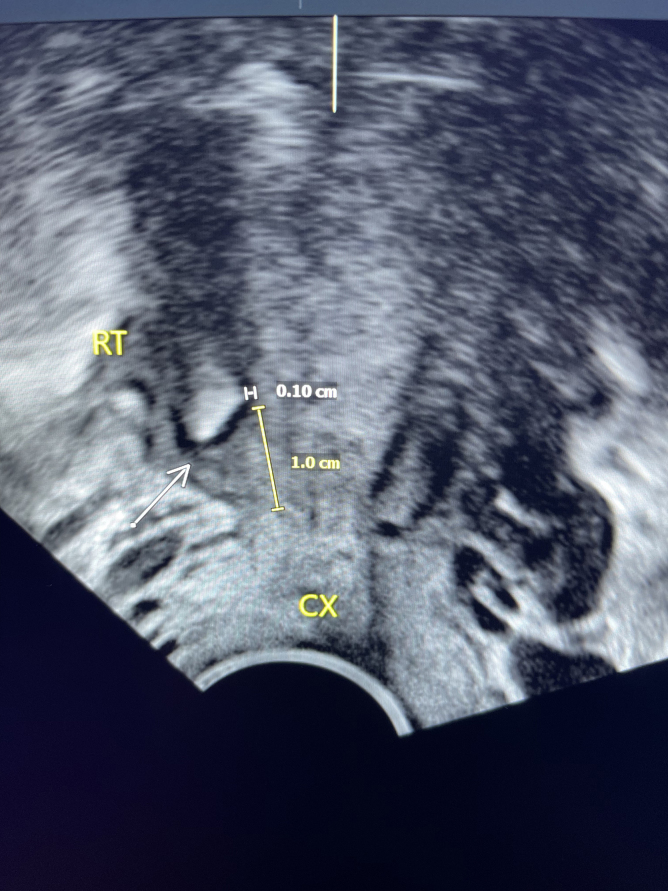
Three-dimensional ultrasound image: arrow points to IUD fragment, 0.10 cm below the endometrial surface and 1.0 cm from the internal os (“CX”), localized to the right myometrium (“RT”).
